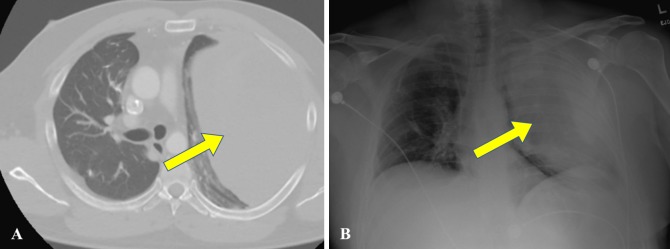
The computed tomography of the chest (A) and x-ray (B) revealing large left-sided hemithorax.
